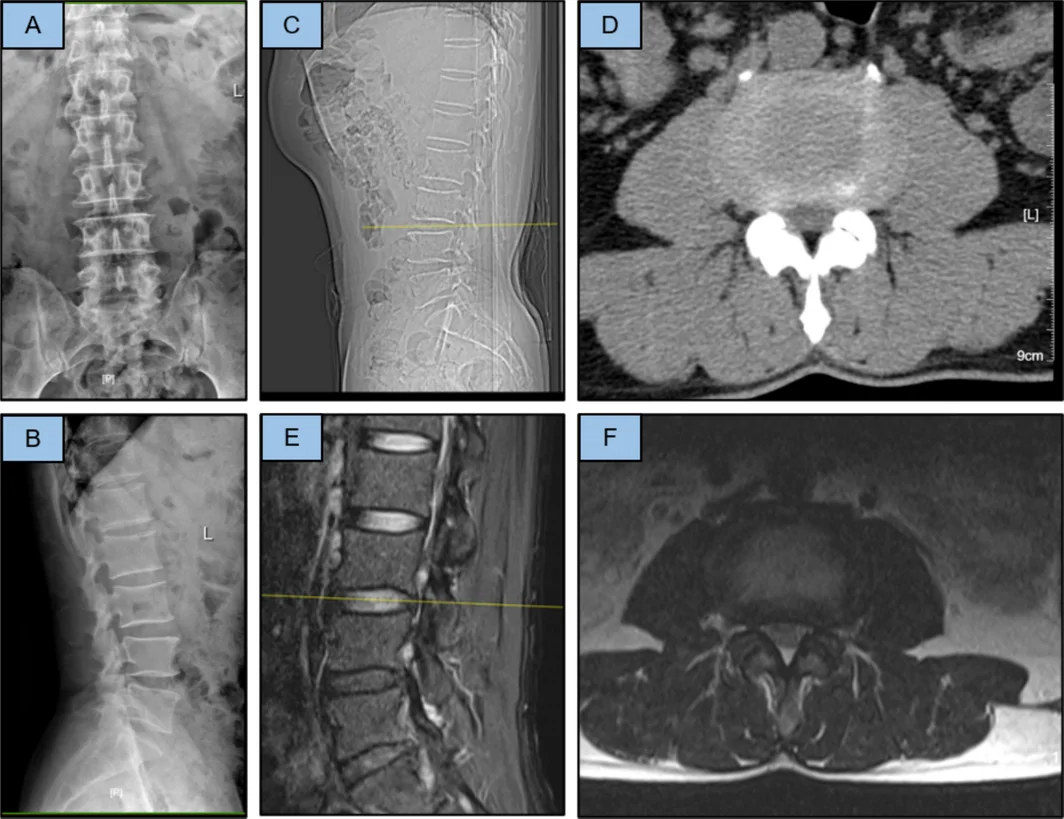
Pre‐treatment radiological assessment of the lumbar spine*. (A, B) Anteroposterior and lateral radiographs showing mild lumbar spondylosis. (C) CT scout view indicating the L3–L4 axial scan plane (yellow line). (D) Axial CT at L3–L4 showing disc bulging and facet hypertrophy. (E) Sagittal T2‐weighted MRI. The symptomatic L3–L4 level (yellow line) shows mild degeneration (Pfirrmann Grade II‐III), contrasting with the severely degenerated L4‐L5 “black disc” (Pfirrmann grade IV). (F) Axial MRI at L3–L4 revealing a right paracentral disc protrusion. This causes mild‐to‐moderate lateral recess stenosis, directly impinging on the traversing right L4 nerve root.
